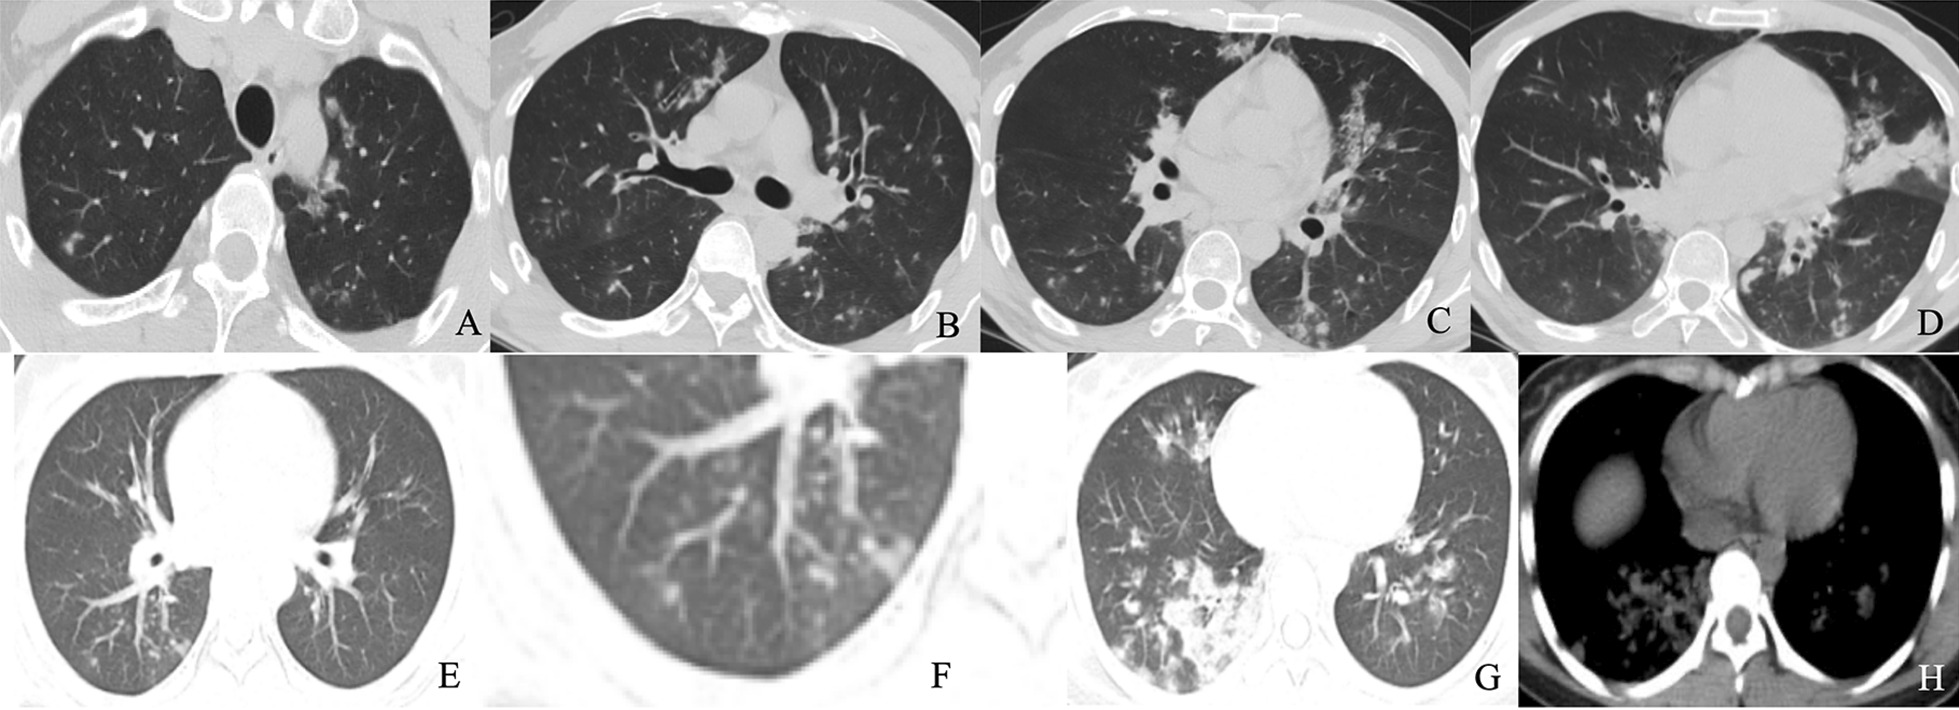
Chest CT findings of influenza pneumonia. Male, 35 years old, 4 days after onset. Multifocal GGO, partial consolidation and consolidation in both lungs (a–d). Feale, 39 years old, 6 days after onset. Multifocal GGO, partial consolidation and consolidation in the right lower lungs (e, g). tree-in-bud (f), and consolidation (h)
